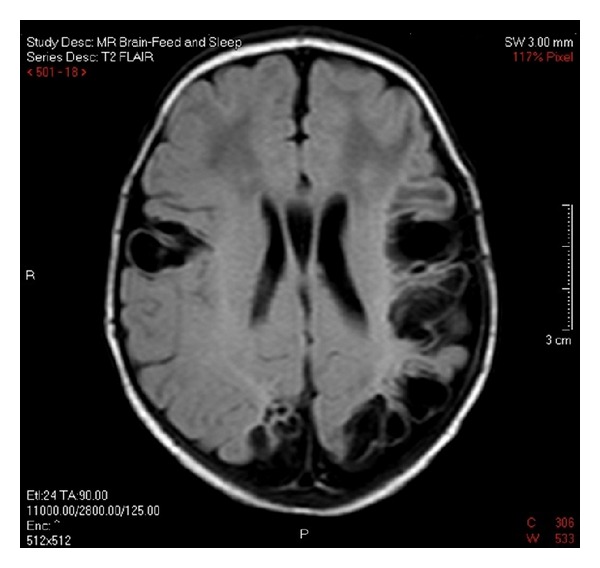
T2-FLAIR imaging four weeks postpartum showing significant lesions at the left parieto-occipital area and to a lesser extent on the right.
